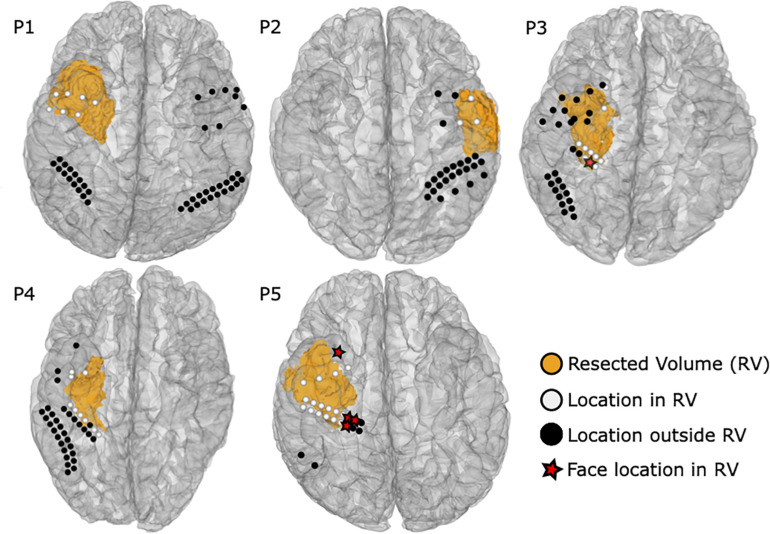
Resected volumes from the post-operative MRI are shown as orange blobs. ECoG and ECS locations are colored white within 10 mm from the resected volume, whereas black labeled ROI locations were considered as distant and thus not relevant to the surgical outcome. Red stars highlight face selective locations in the resected volume.
